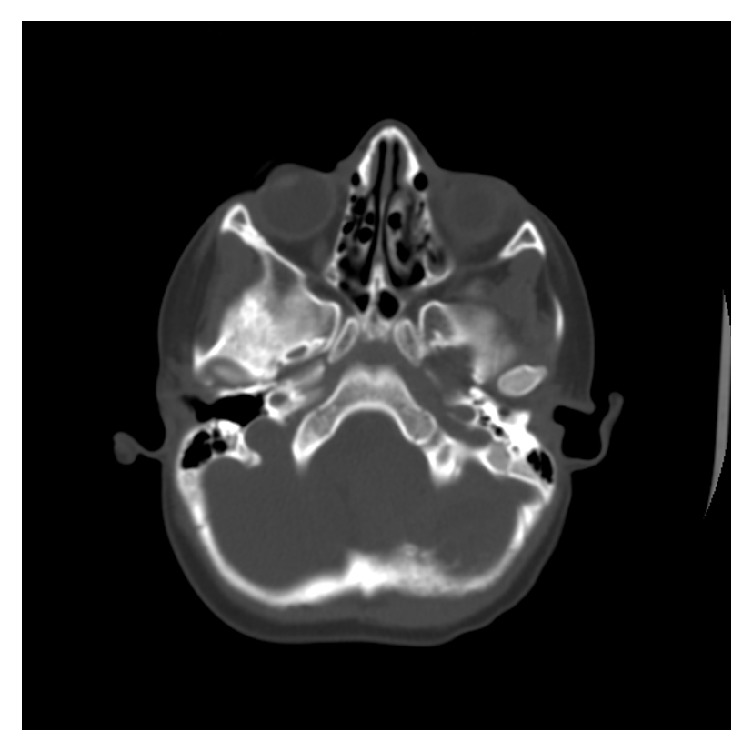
Axial CT scan bone window shows loss of the inner skull table and cortical outline with permeative bone reaction indicating bone destruction.
